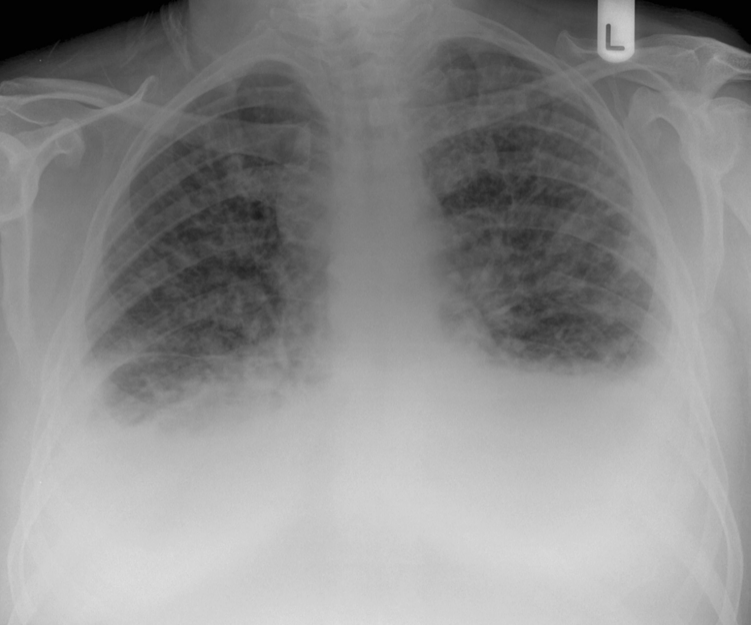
Chest X-ray: bilateral pleural effusions and extensive interstitial reticulo-nodular shadowing.
